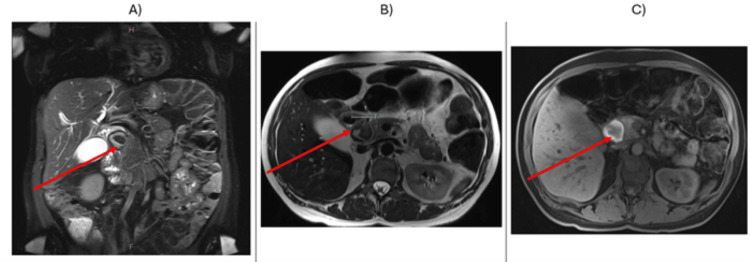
MR imaging of the biliary tract and pancreatic lesion (red arrow) in A) coronal T2 phase with evidence of biliary obstruction, B) axial T2 phase and C) T1 hyperintensity suggestive of protein-rich fluid within the cyst – highly suggestive of blood products.
